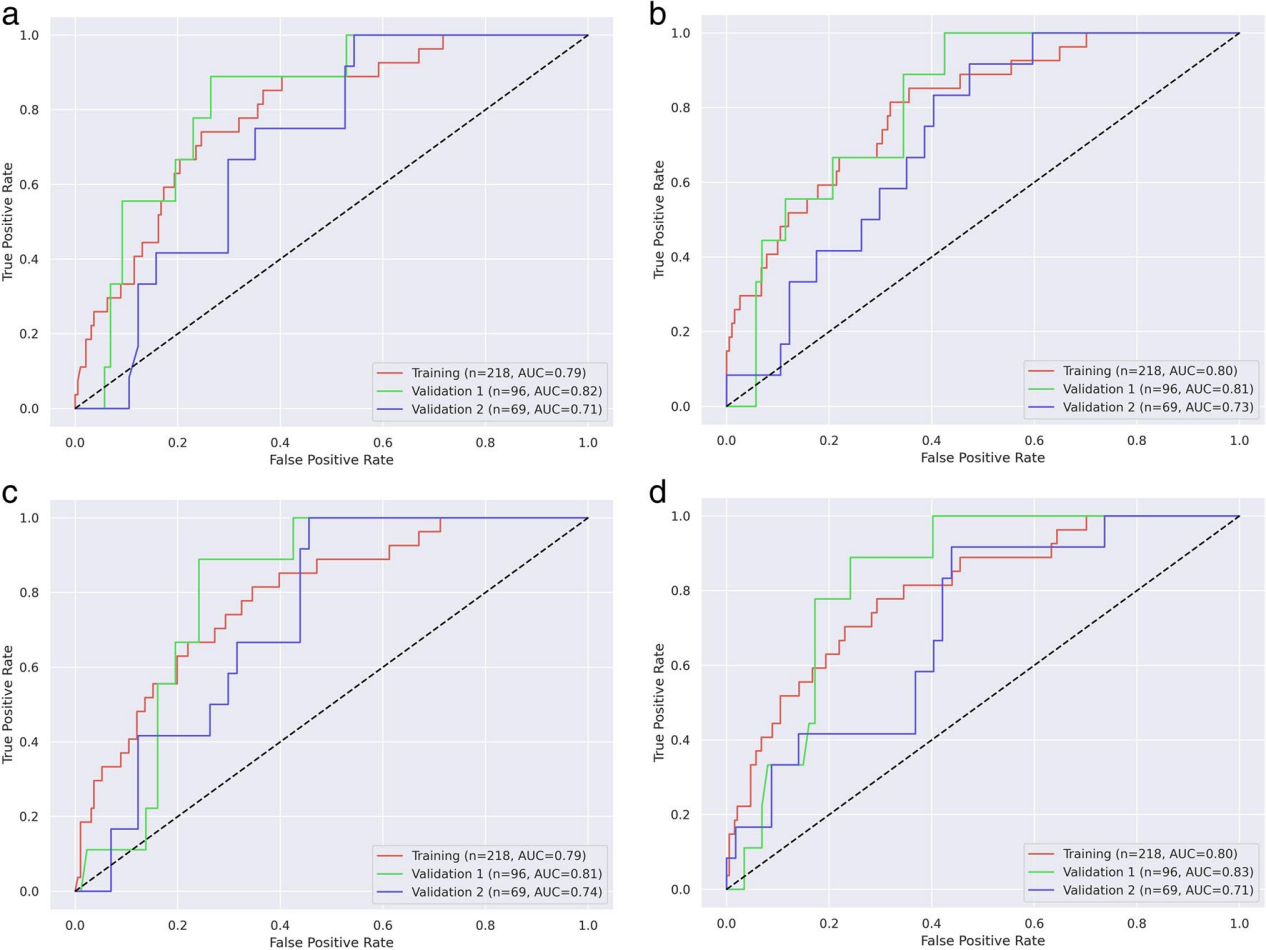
Comparison of receiver operating characteristic curve in a model trained with non-contrast-enhanced CT images alone and different phases of contrast-enhanced CT images. a Model trained with non-contrast-enhanced CT images; b model trained with arterial phase on contrast-enhanced CT images; c model trained with venous phase on contrast-enhanced CT images; d model trained with delayed phase on contrast-enhanced CT images. AUC, area under the receiver operating characteristic curve
